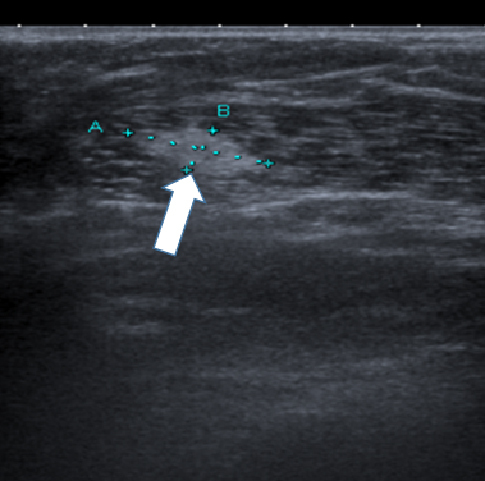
An ultrasound scan of the patient’s chest wall taken to investigate the presence of a palpable lump, later diagnosed as a lipoma. The absence at this time of the calcified lesion later visualized on CT scan is noteworthy.
